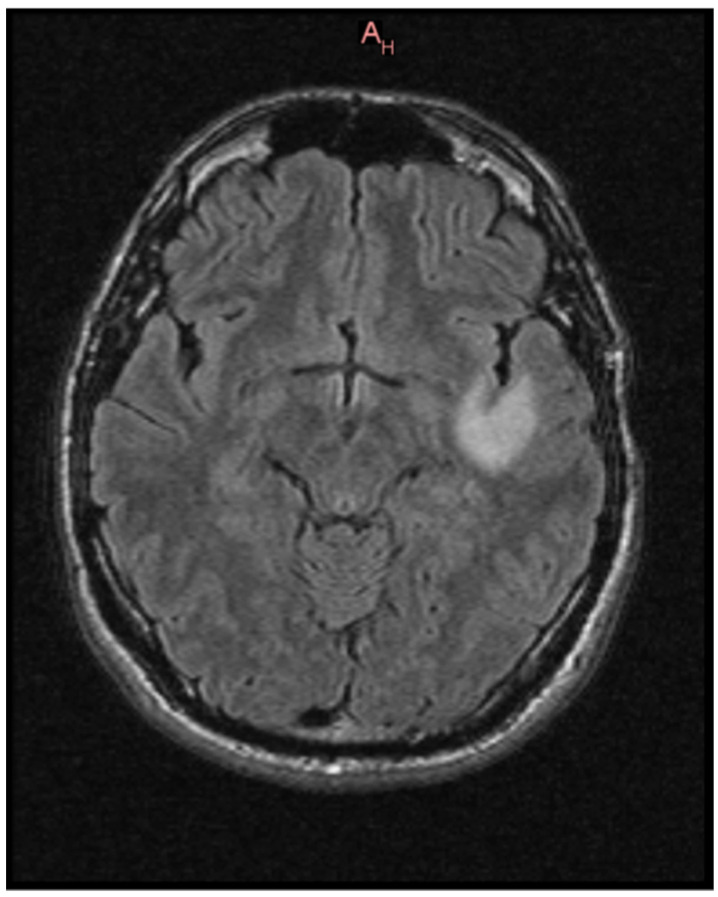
Brain MRI of a left insular–temporal lesion in axial section.
